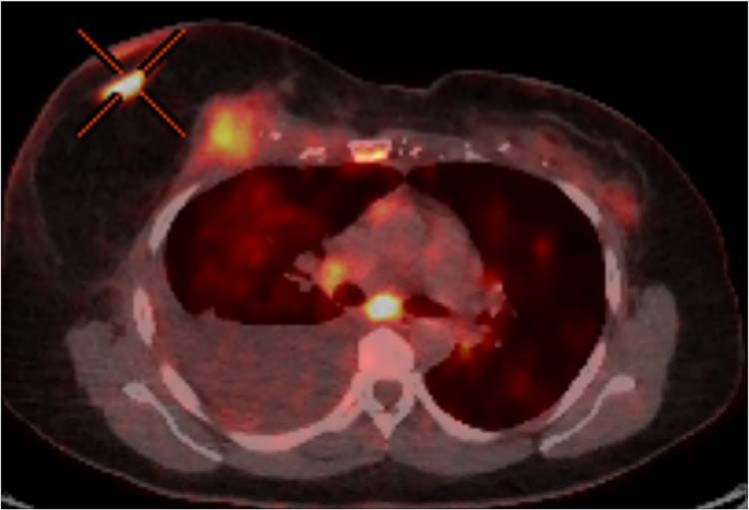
PET-CT axial slice showing both superficial and deep components of the local recurrence with associated malignant pleural effusion from direct extension to the pleural cavity.
